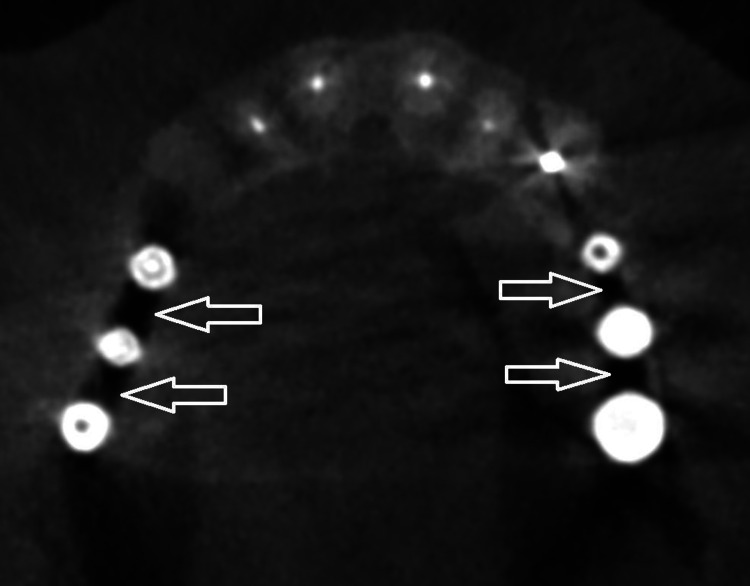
Dark streaks between dental implants in cone-beam computed tomography (type of beam hardening artifacts) Image credit: The authors of the current study.
